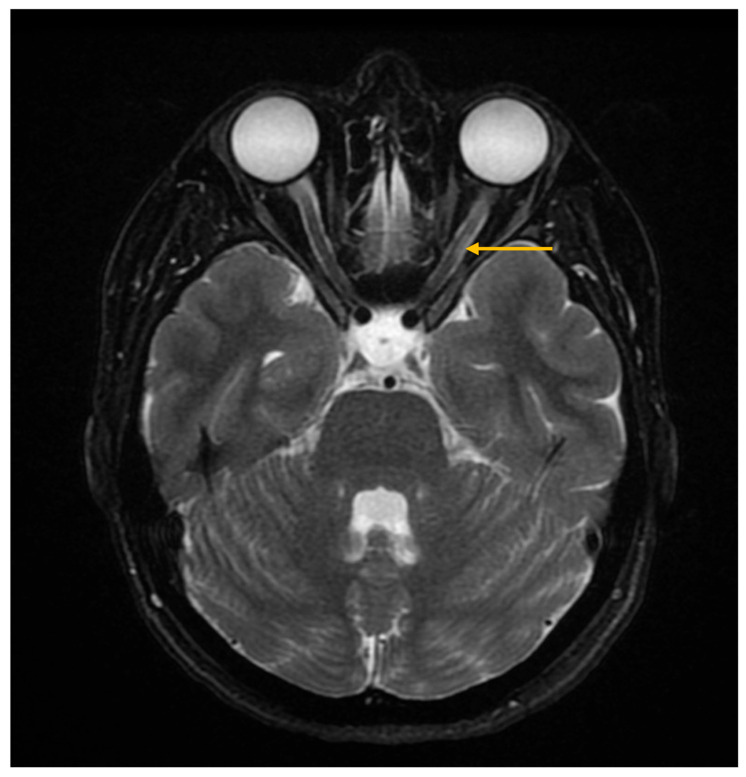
Magnetic resonance imaging (MRI) of the brain and orbits, axial T2-weighted short tau inversion recovery (STIR) sequence. The image demonstrates hyperintensity of the left optic nerve (arrow), consistent with optic neuritis.
